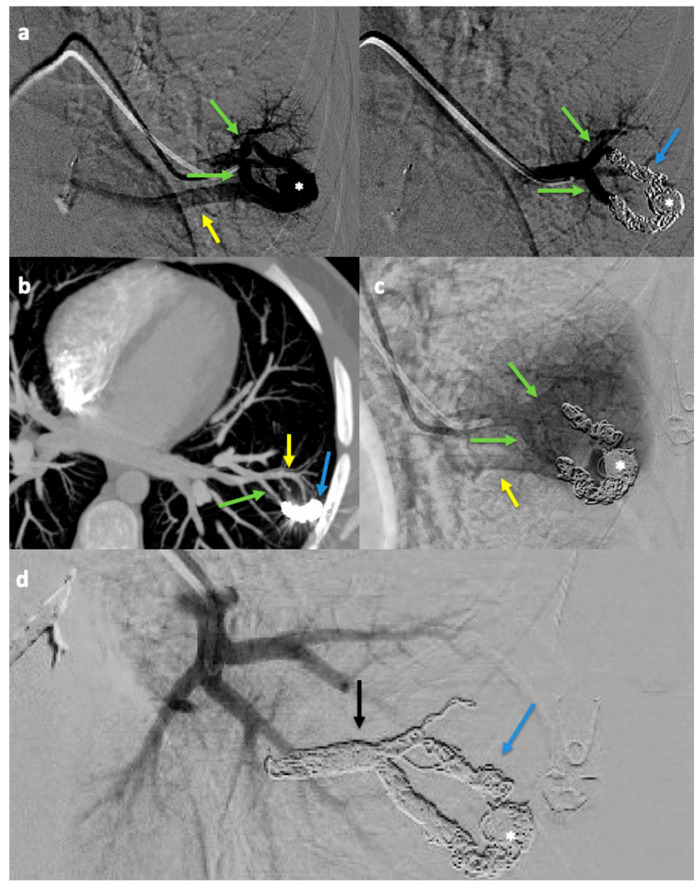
Treatment failure and retreatment after nidus-plus-feeding artery (NiFA) coil embolization (a) Digital subtraction angiography (DSA) before (left) and after (right) coil embolization of the dominant feeding arteries (green arrows) and nidus (asterisk) using Interlock-18 standard 8 mm × 20 cm, three 6 mm × 20 cm, one 4 mm × 12 cm, one 3 mm × 12 cm, one interlock-18 soft 4 mm × 12 cm, and three 3 mm × 14 cm microNester coils (blue arrow) in a left lower lobe PAVM. The draining vein (yellow arrow) is seen only prior to embolization. (b) Axial maximum intensity projection (MIP) CT angiography obtained 14 months after the procedure demonstrates residual flow through the coil pack (blue arrow) and draining vein (yellow arrow) distal to a dominant FA (green arrow), consistent with treatment failure due to recanalization. (c) Digital subtraction angiography 5 years after the initial procedure demonstrates recanalization of the nidus (asterisk) and draining vein (yellow arrow) distal to the dominant feeding arteries (green arrows). (d) Following coil embolization of the two dominant feeding arteries proximal to the pre-existing coil pack (blue arrow) using a Ruby 3 mm × 15 cm standard coil and two Ruby 60 cm packing coils (black arrow), the draining vein and nidus (asterisk) no longer opacify.
